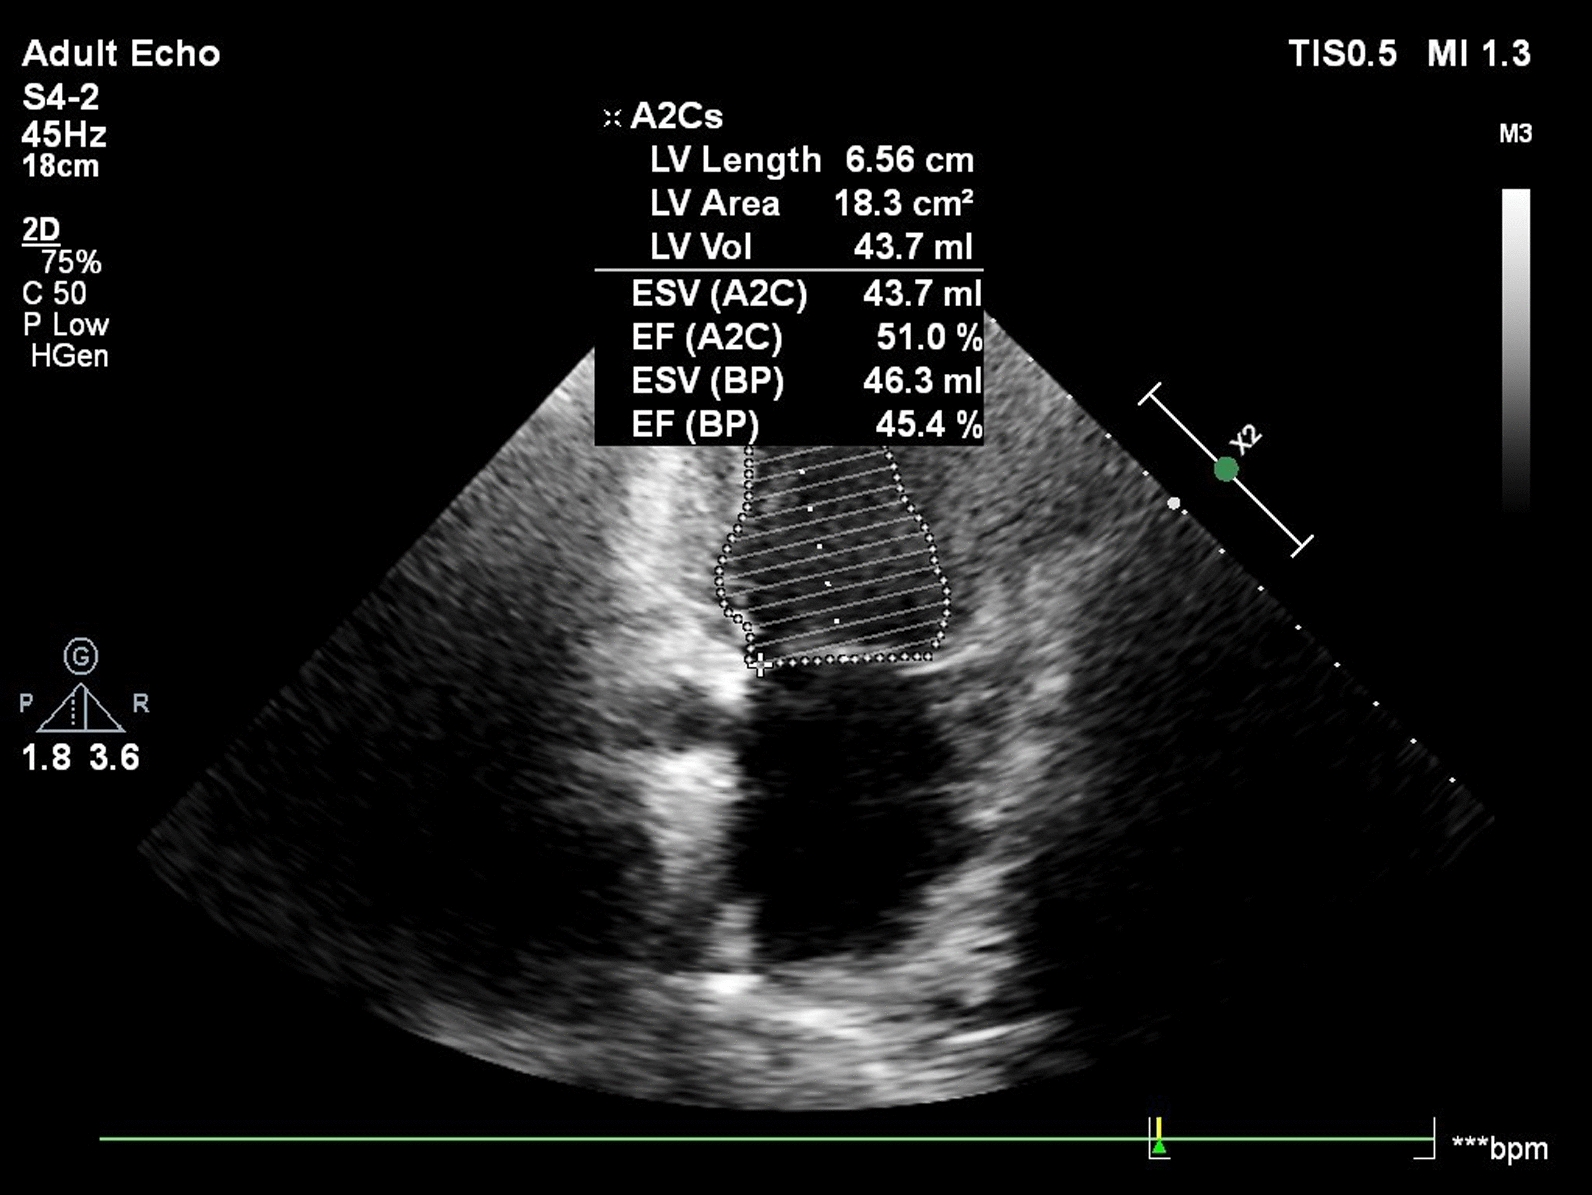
Echocardiography showing ischemic heart disease with mild impairment of the left ventricle systolic function and a hypokinetic mid, basal inferior, and inferior lateral segments
